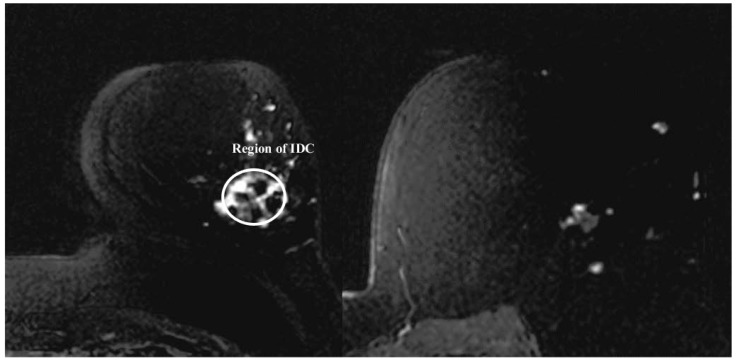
MRI scan of Patient 3 (from [24]). For Figure 6, IEEE License received. License number 4274890619942.
